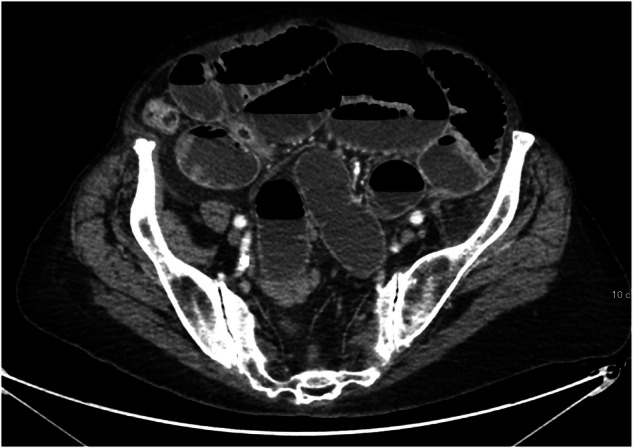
CT image of the lower abdomen with dilated small bowel loops consistent with obstruction. GPT-4V correctly identified the modality and the anatomical region but misclassified the image as normal
